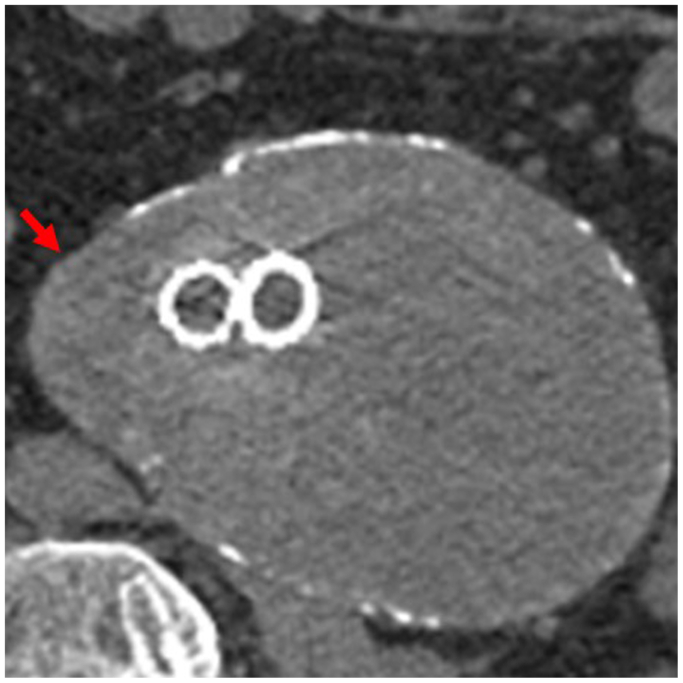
Noncontrast surveillance computed tomography (CT) scan demonstrating sac expansion to 8.7 × 10.2 cm with a sac volume of 516 mL. In this study, there was increased focal outpouching of the right aspect of the aneurysm sac (*red arrow*) compared with the prior study, concerning for instability.
